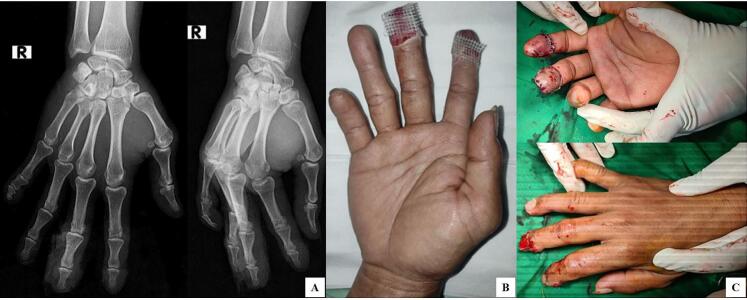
(A) X-ray examination of the right manus showed no fracture; (B) wound dressing; (C) postoperative view after FTSG application.
